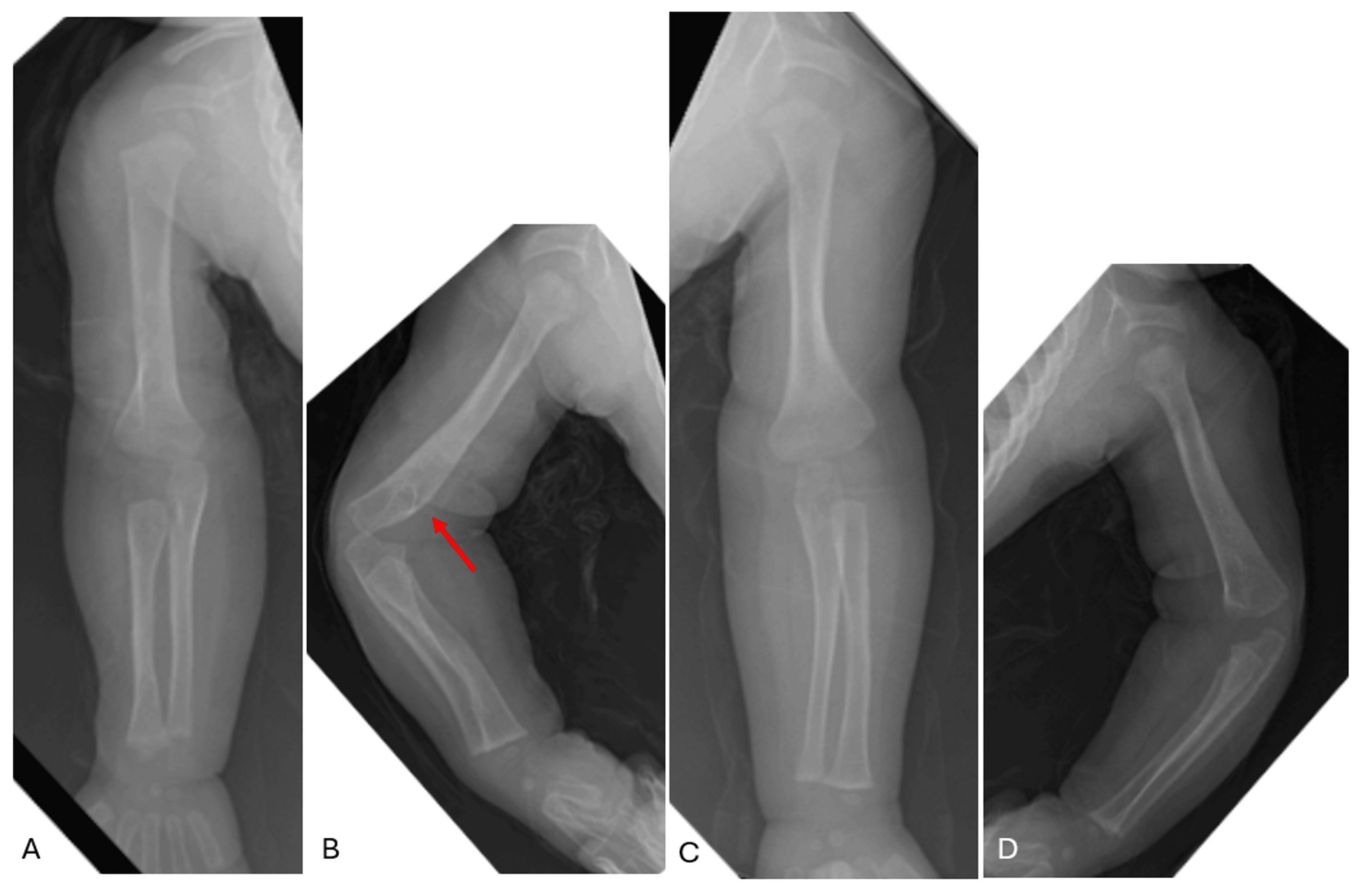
Right anteroposterior (A), right lateral (B), left anteroposterior (C), and left lateral (D) upper extremity radiographs one year of age. The long bones appear normal with no periosteal reaction. Red arrow denotes the mild procurvatum deformity of the right distal humerus.
